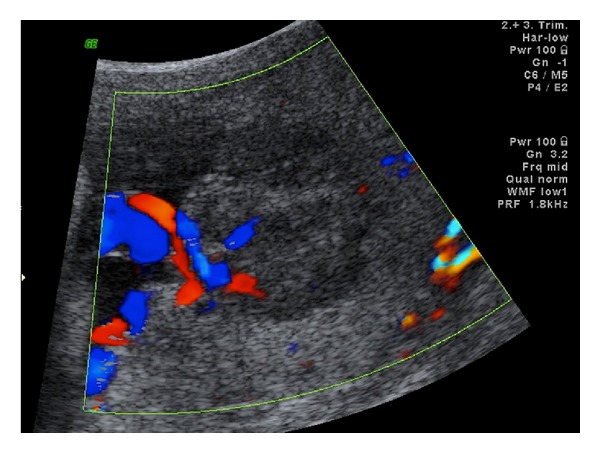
The color Doppler ultrasonography of Case 2 showing the typical place of the tumor next to the umbilical cord insertion.
